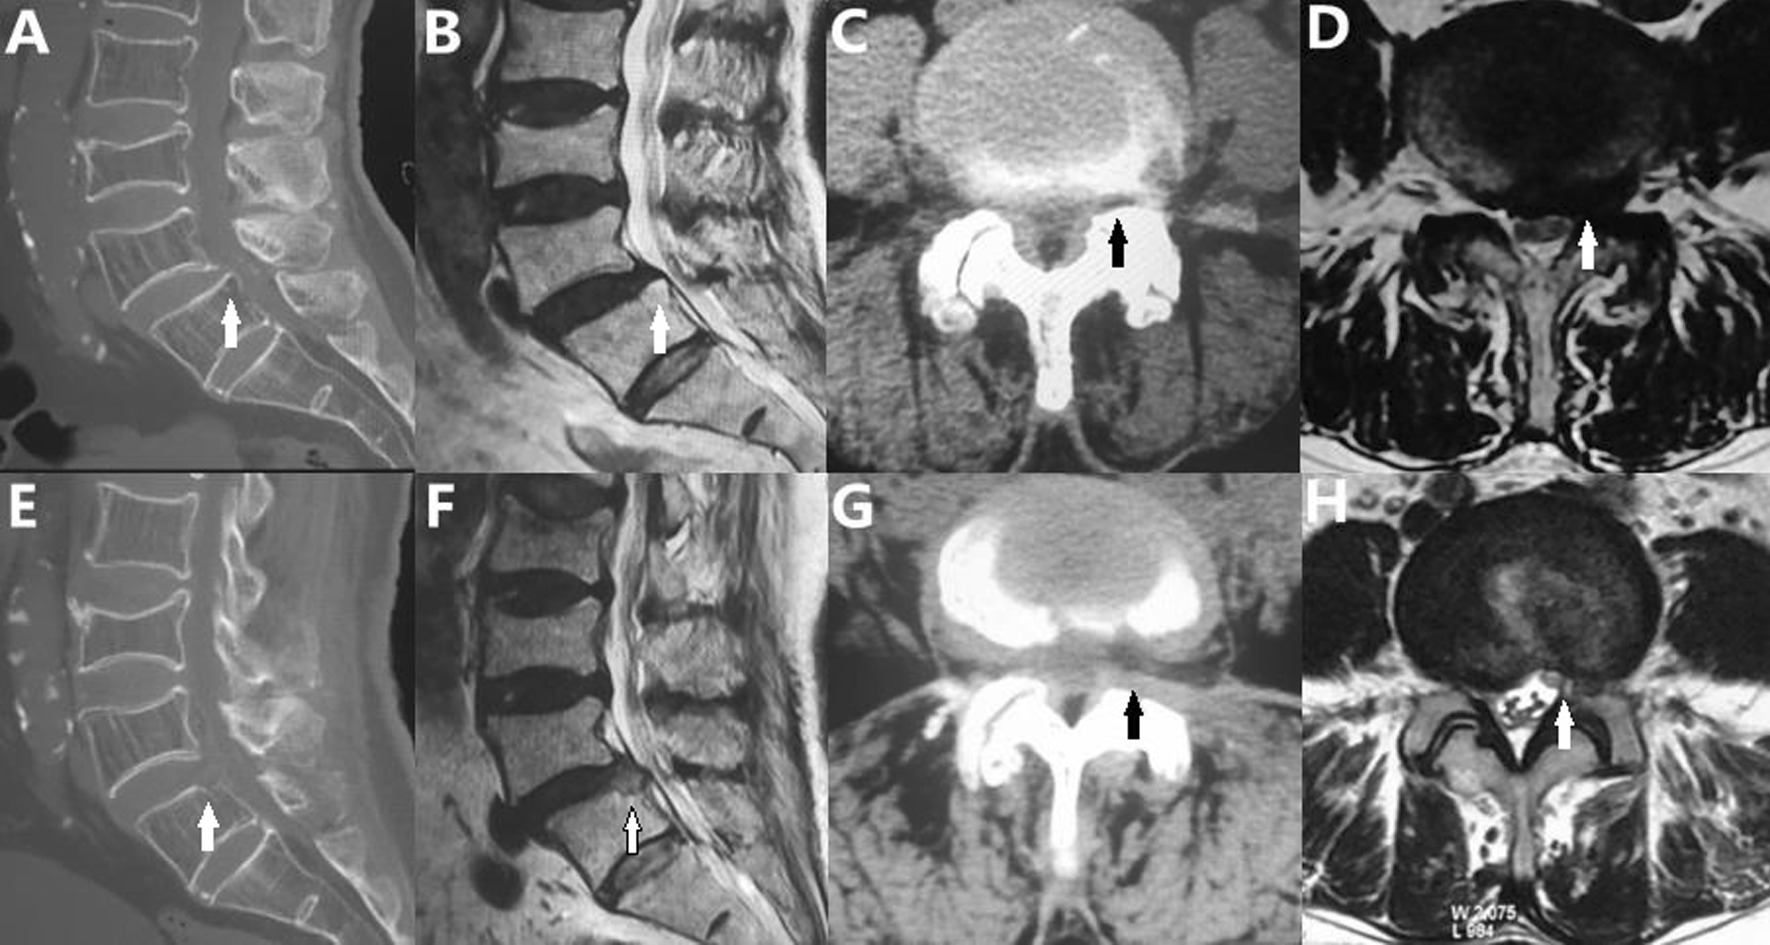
Pre- and postoperative CT and MRI. A–D The superior endplate of L5 before surgery and lateral recess stenosis. E, F The protruded vertebral bone was removed, and the original spinal canal shape was restored. The ventral and dorsal edges of the spinal canal became smooth and continuous. G, H The lateral recess was enlarged
